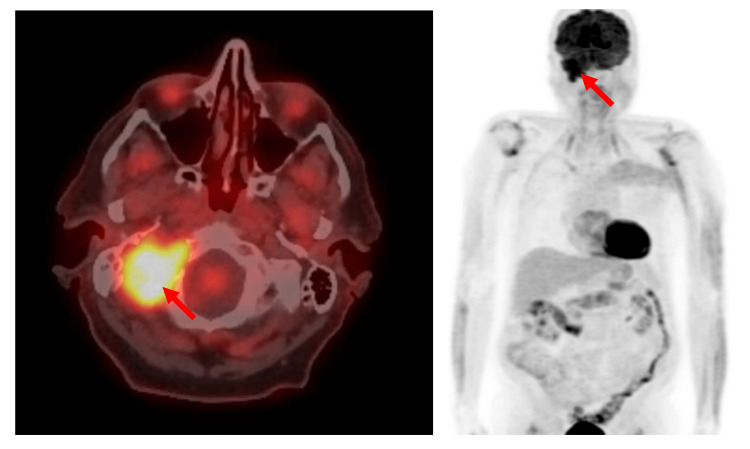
Positron emission tomography (PET) staging at baseline. Left hand side: axial image of the head displayed in “hot-iron” showing intense metabolic activity of the right jugular foramen plasmacytoma. Right hand side: coronal image of the body showing the solitary right jugular foramen plasmacytoma in the absence of radiographic evidence of systemic disease.
